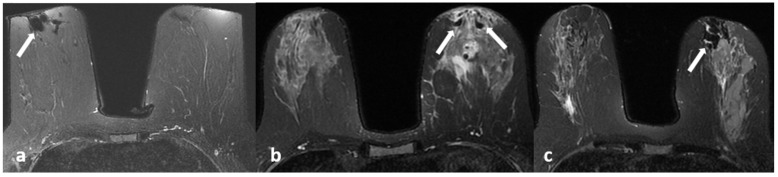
(a–c) Fat containing dilated ducts (arrows) on fat-suppressed T2-weighted images in three different patients.
